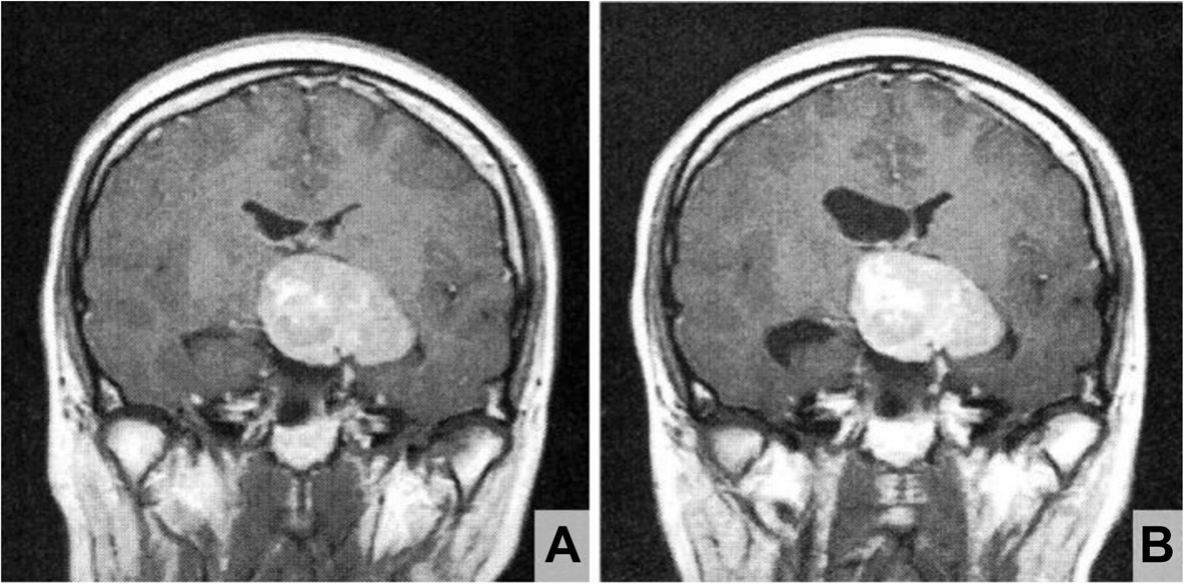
Pretreatment (a) and preoperative (b) coronal T1-weighted magnetic resonance images with contrast (gadolinium). Prior to surgery, slight dilatation of right lateral ventricle evident (b), compared with baseline status (before cabergoline therapy) (a)
